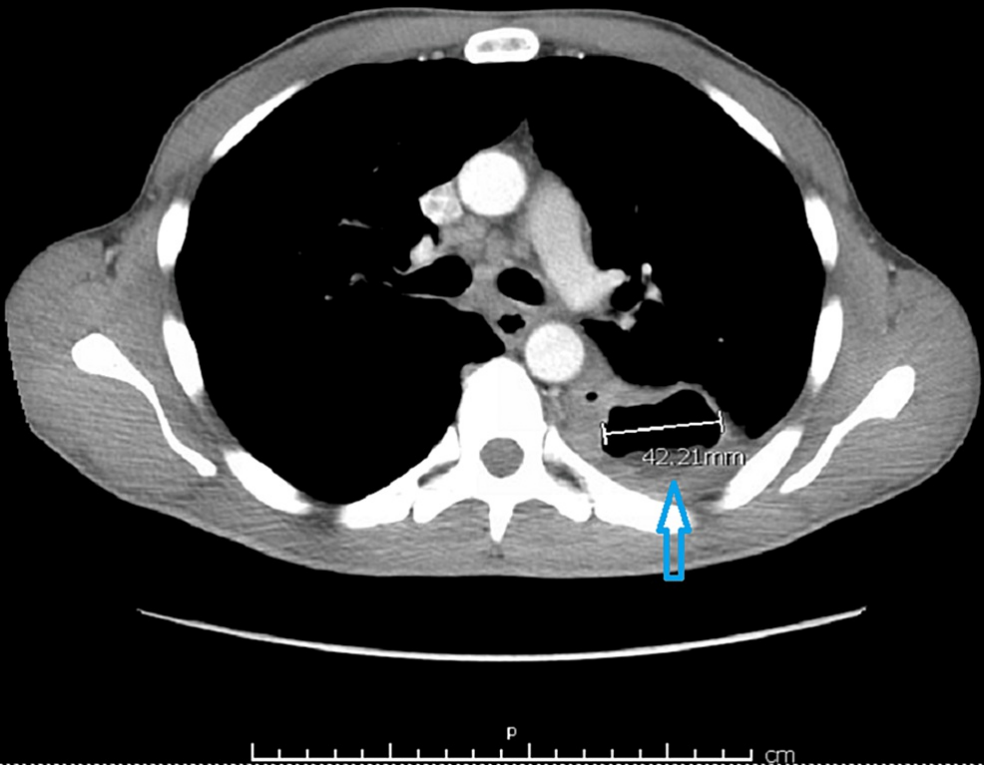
Computed tomography (CT) scan of the chest showing 4.2x2.0 cm interval cavitary process compatible with lung abscess as shown with blue arrow.
